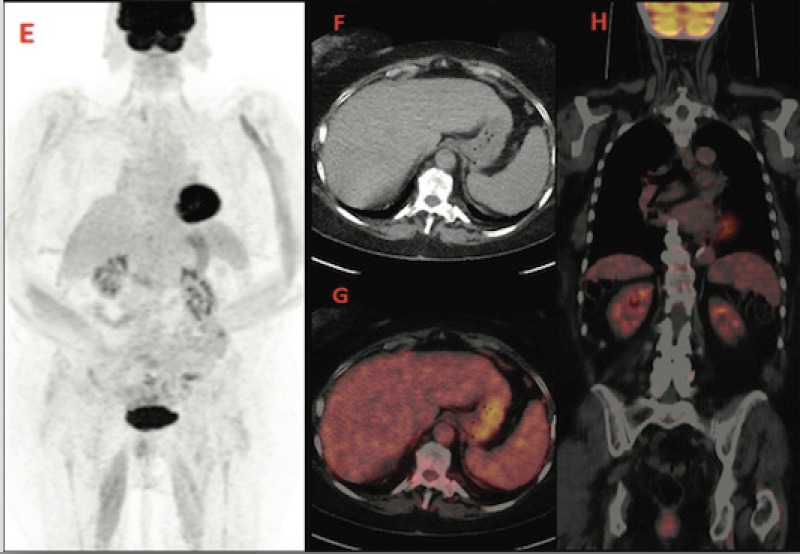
PET-CT after treatment that shows a rapid decrease of FDG uptake.
